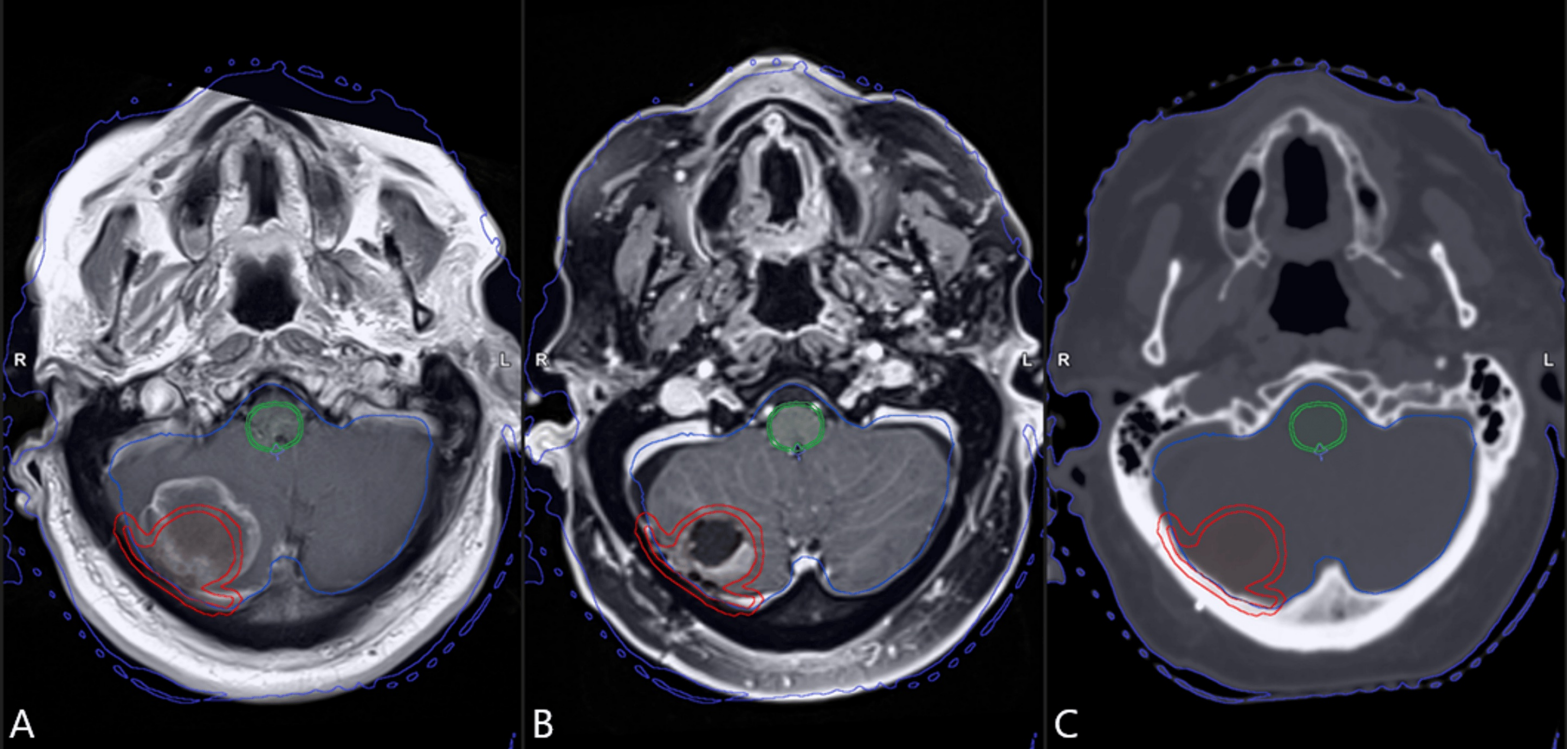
Pre- and post-operative imaging scans for a typical cavity SRS patient. (A) Pre-operative T1-weighted post-contrast diagnostic MRI scan, (B) post-operative T1-weighted 3D fast gradient echo sequence post-contrast planning MRI scan, (C) planning CT scan with 1 mm slice separation. In all the images, the tumour cavity outline (extended for pre-operative dural contact) and the planning target volume (PTV), grown with a 2 mm margin, are shown in red; the brainstem and planning organ at risk volume (PRV), grown with a 1 mm margin, are shown in green.
